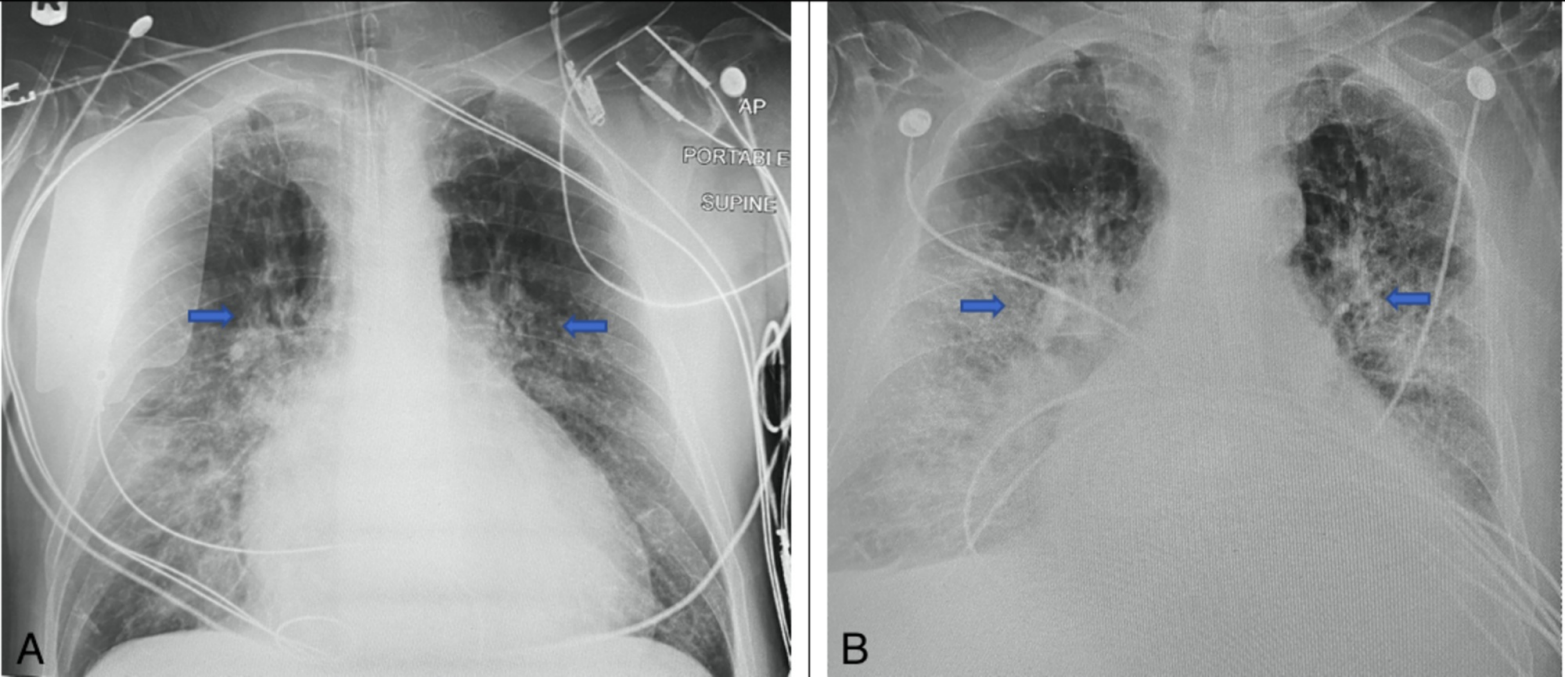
X-rays of chest from anteroposterior view from day 1 (A) and day 2 (B) respectively. The arrows in the above image indicate bilateral perihilar and infrahilar opacities with predominant involvement on the right side.
